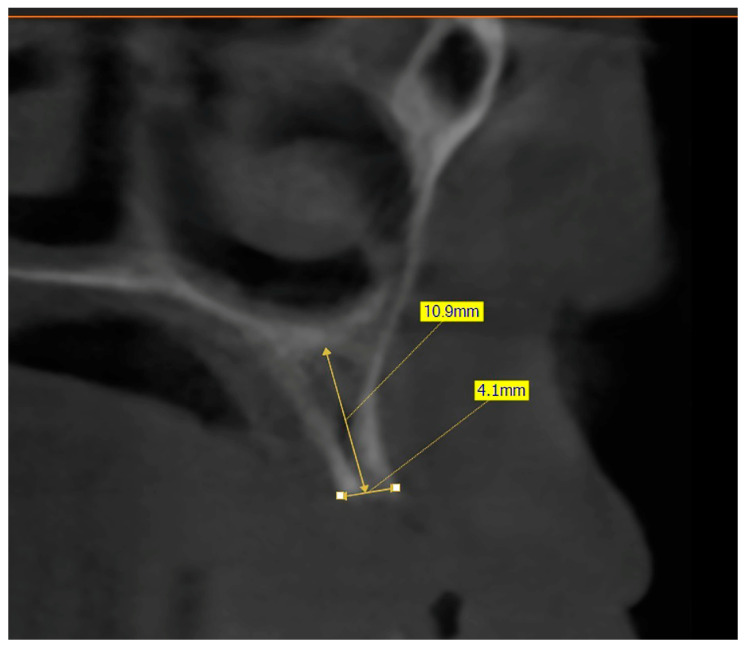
Bone measurement on a cross-sectional image—CBCT (Green X™ 12^®^, Vatech, Prague, Czechia).
